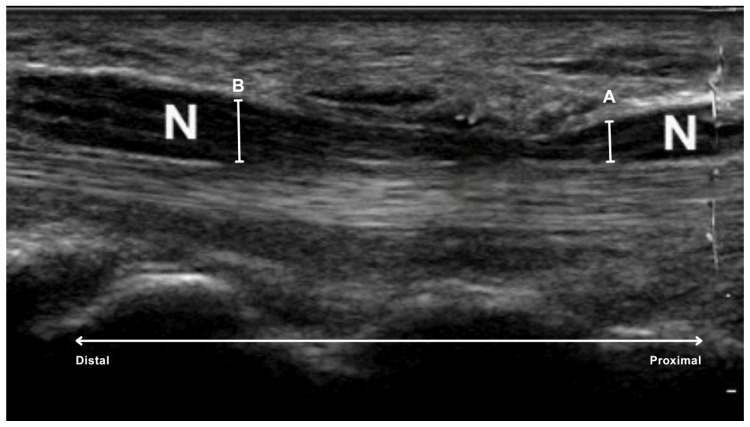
Sonographic measurement sites of the median nerve (N) in carpal tunnel syndrome. A, measuring point at the level of the first carpal row; B, Measuring point under the flexor retinaculum.
